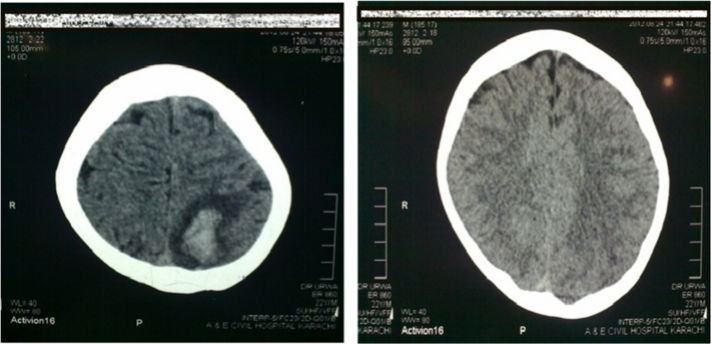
Computed Tomographic (CT) scan of brain showing hemorrhagic infarct.
